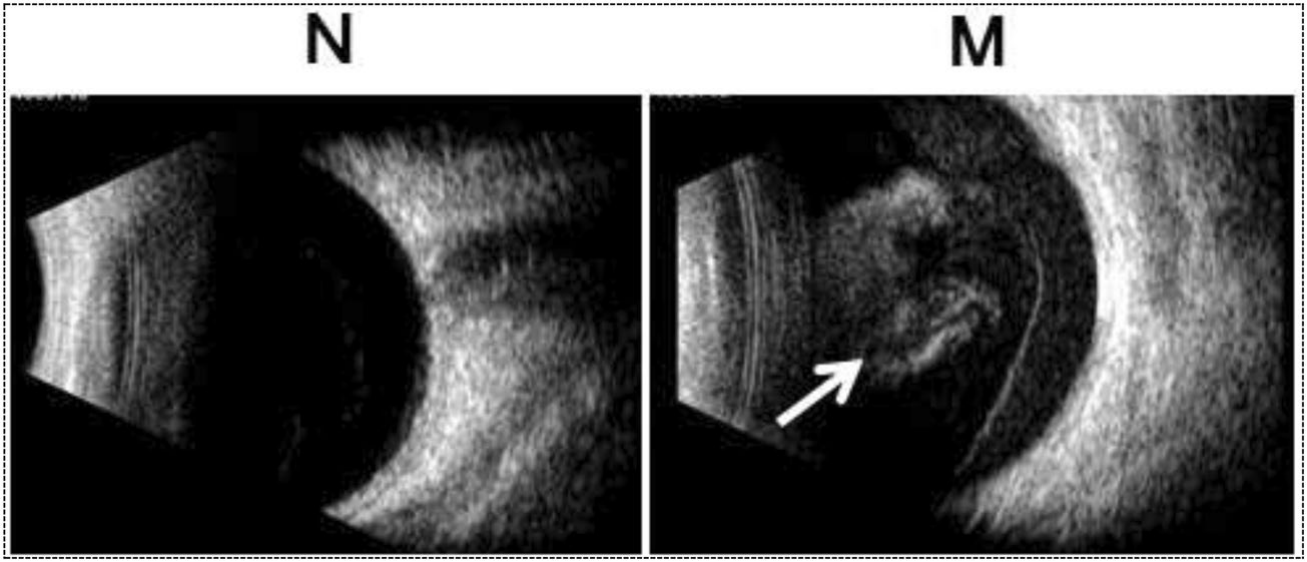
Typical pictures of the B-scan ultrasonography in eyes without vitreous hemorrhage and eyes with vitreous hemorrhage. An obvious vitreous hemorrhage was shown in the typical pictures of the B-scan ultrasonography in eyes with vitreous hemorrhage, compared to that of the normal eye. N: eyes without vitreous hemorrhage; M: eyes with vitreous hemorrhage; The white arrow: the vitreous hemorrhage.
